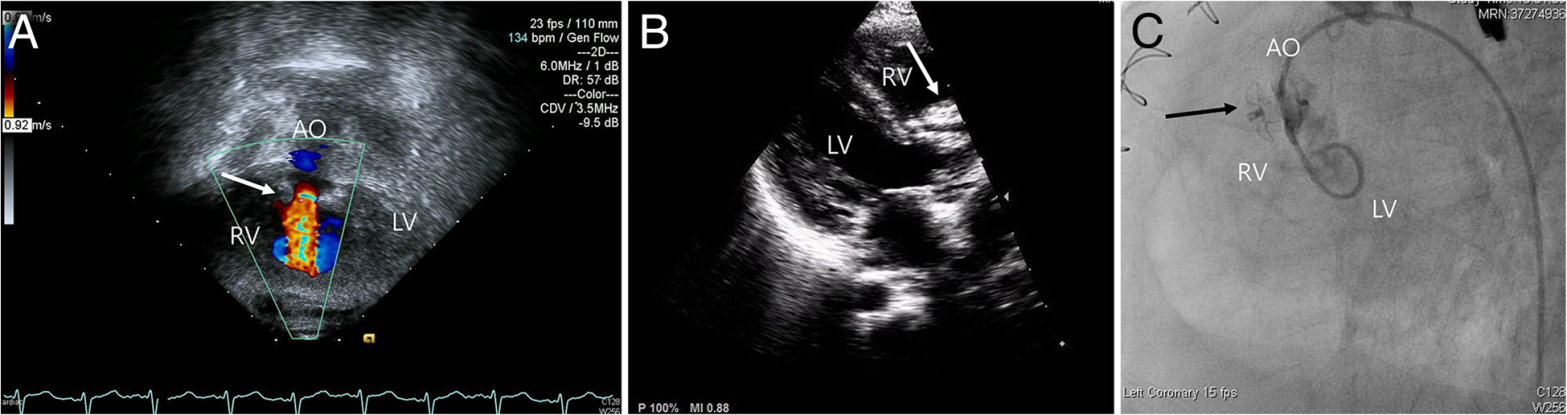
Device closure of VSD fenestration with Coccoon VSD occlude a, Transthoracic echocardiography and color Doppler showed VSD through patch fenestration (white arrow). b Two dimensional echocardiography showed implanted Coccoon device successfully (white arrow). c A good device position (black arrow) and immediate minimal leakage was proven on LV angiography. LV, left ventricle, AO, aorta, RV, right ventricle, VSD, ventricular septal defect
